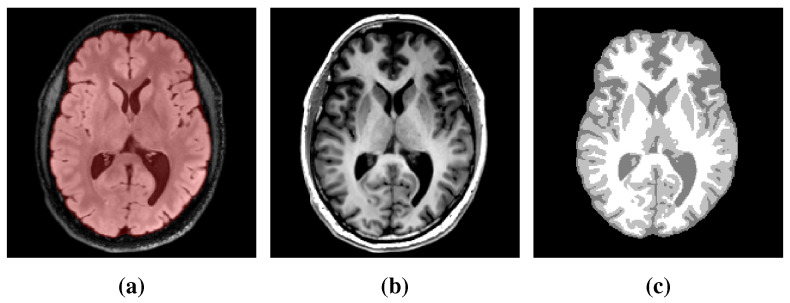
Example of processed MR data. (a) Axial slice of the T2 FLAIR image with superimposed segmented brain mask (red). (b) Corresponding slice of the T1 BRAVO image. (c) Segmented brain map obtained with the MICO algorithm [38] followed by manual corrections.
